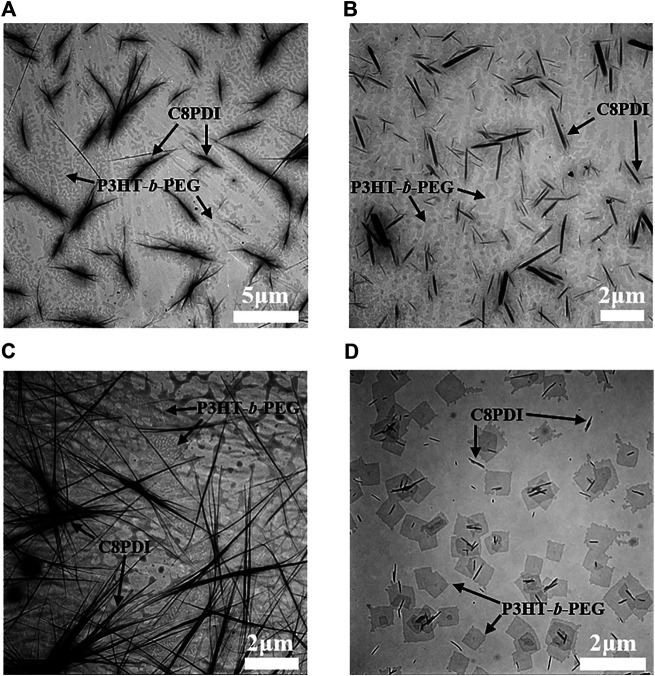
TEM images of P3HT-*b*-PEG and C8PDI drop-cast from *o*-DCB solution (10:1) before (A) and after (B) ultrasound treatment. TEM images of P3HT-*b*-PEG and C8PDI drop-cast from Tol solution (10:1) before (C) and after (D) ultrasound treatment.
